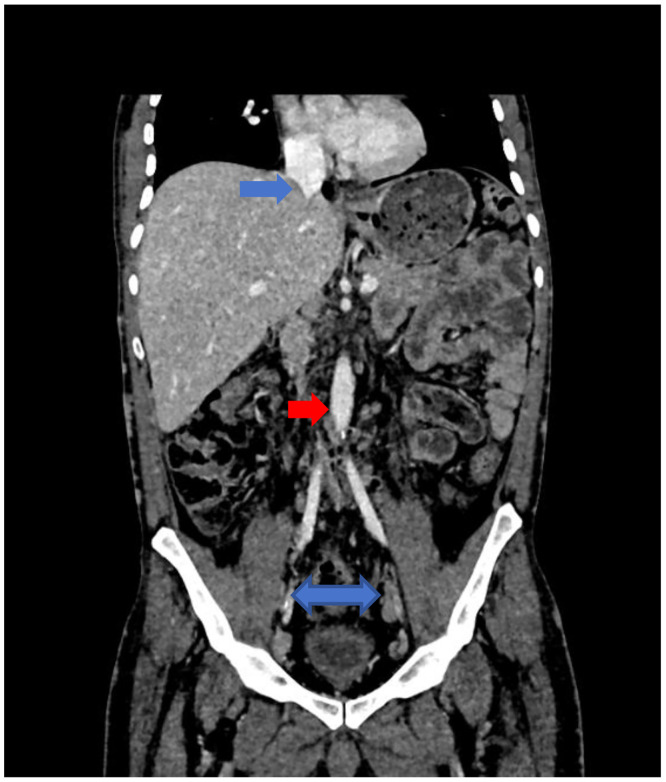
Abdomino-pelvic CT scan: Coronal plan through the IVC showing partial agenesis of intrahepatic segment and complete agenesis of infra-hepatic segment (blue arrow), normal abdominal aorta (red arrow) and iliac veins (blue double-headed arrow).
